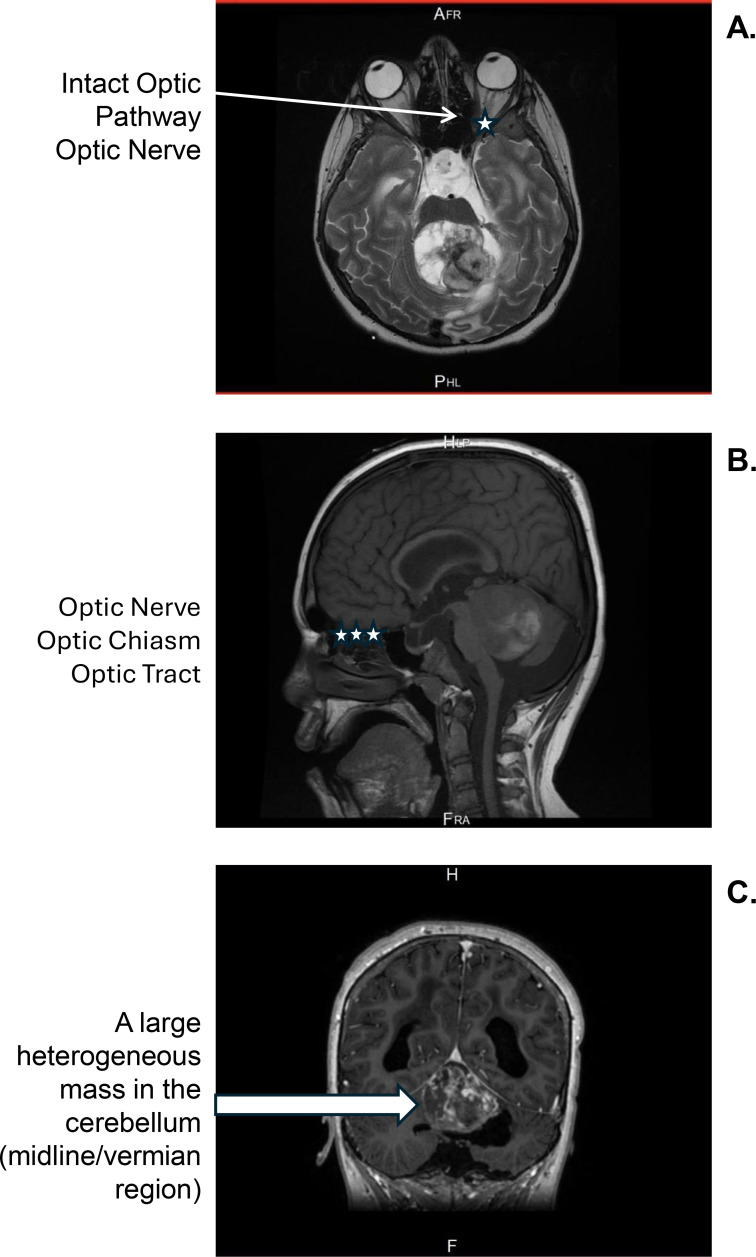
Pre-operative post-contrast MRI of the brain. (A) Axial view demonstrating the intact optic nerve (star) with no tumor involvement of the anterior visual pathway; arrow indicates the posterior fossa tumor. (B) Sagittal view with the anterior visual pathway structures identified (stars): optic nerve, optic chiasm, and optic tract. These structures appear intact with no evidence of tumor extension or compression, confirming the anatomical separation between the posterior fossa tumor and the anterior visual pathways. (C) Coronal view demonstrating a large heterogeneous mass in the cerebellum (arrow) with compression of the surrounding cerebellar tissue and the fourth ventricle, consistent with recurrent pilocytic astrocytoma causing obstructive hydrocephalus. MRI, magnetic resonance imaging.
